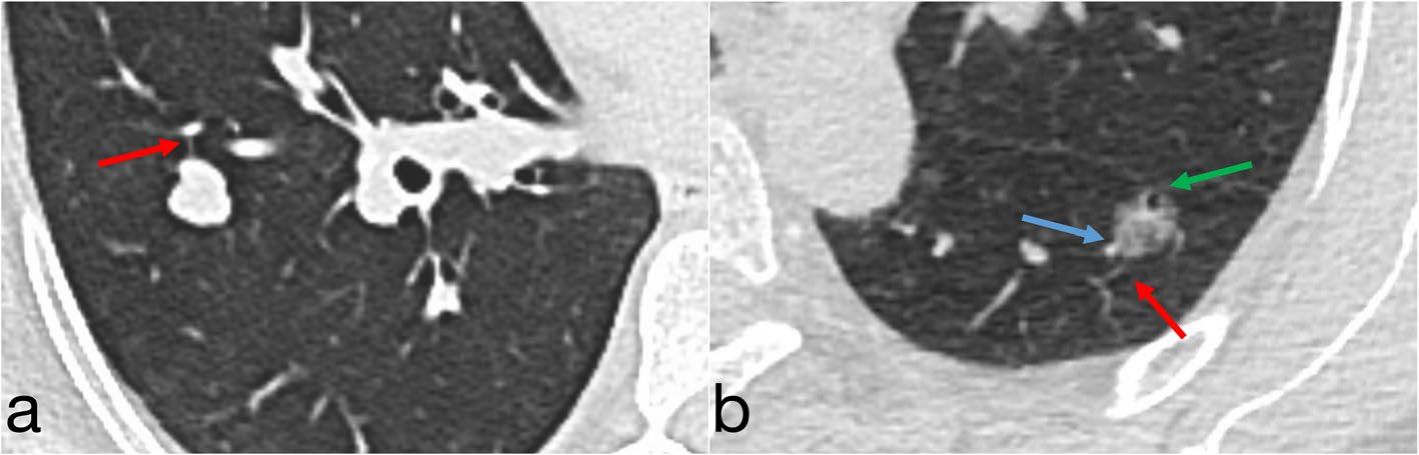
Computed tomography image shows vascular convergence (blue arrows), pleural retraction (green arrows), and spiculation (red arrows). a a solid nodule with an average diameter of 1.1 cm in the right lower lobe of a 54-year-old woman’s lung and pathologically confirmed to be benign PN; b a part-solid nodule with an average diameter of 1.1 cm in left lower lobe of 58-year-old woman’s lung and pathologically confirmed to be malignant PN
